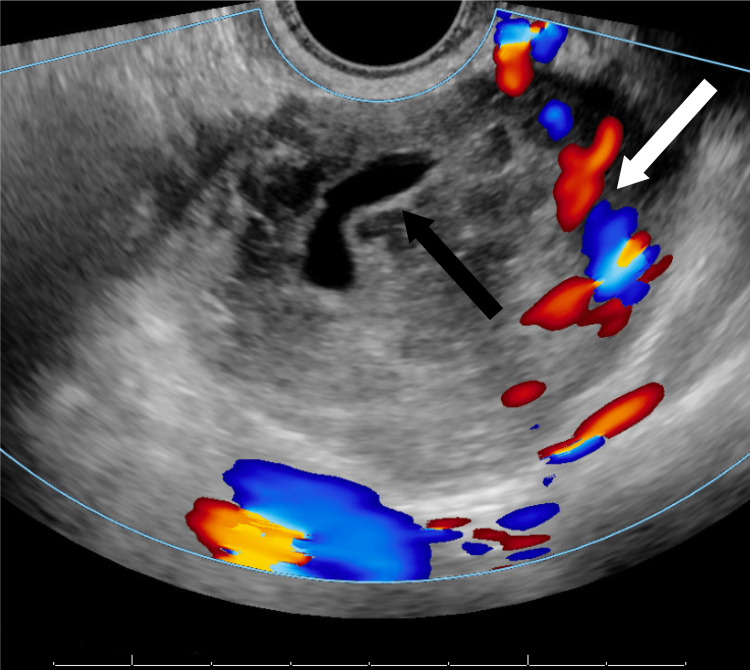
A transvaginal ultrasound depicting an ovarian ectopic pregnancy. The black arrow demonstrates a hyperechoic ring. The white arrow demonstrates a "ring of fire."
